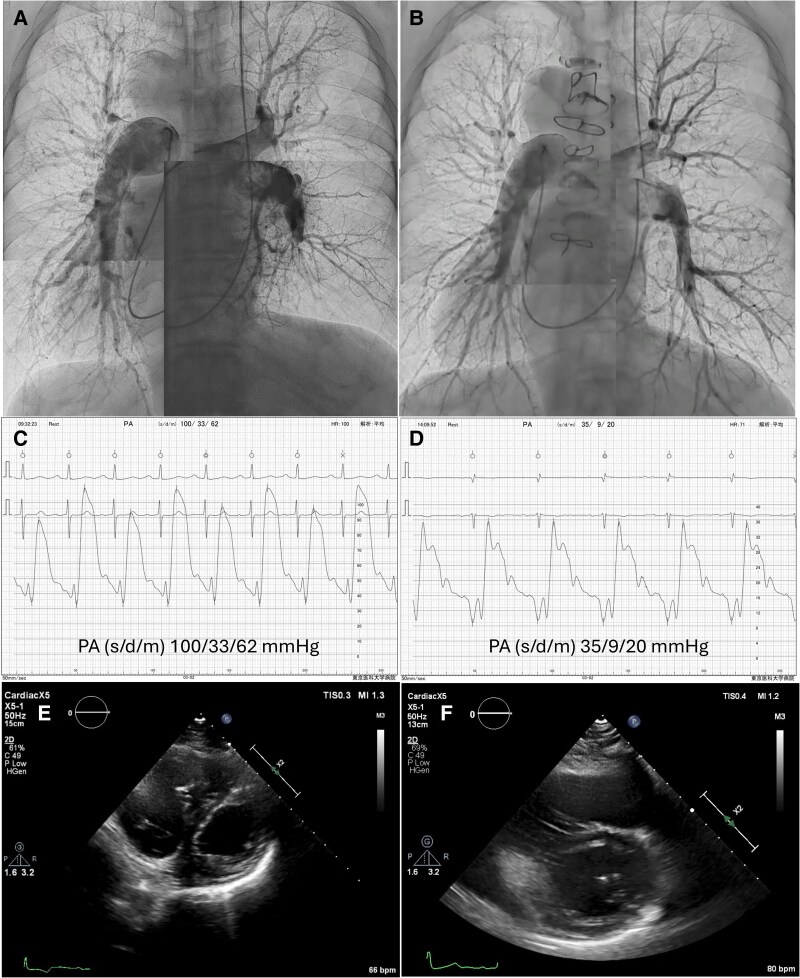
Pulmonary angiography, curve of pulmonary arterial pressure, and thoracic echocardiography before and after two balloon angioplasties and pulmonary endarterectomy. (*A*) Initial pulmonary angiography. (*B*) Pulmonary angiography after two balloon angioplasties and pulmonary endarterectomy. (*C*) Initial curve of pulmonary arterial pressure. (*D*) Curve of pulmonary arterial pressure after two balloon angioplasties and pulmonary endarterectomy. (*E*) Initial short-axis view in thoracic echocardiography when the patient lay down on right side. (*F*) Short-axis view in thoracic echocardiography after two balloon angioplasties and pulmonary endarterectomy. PA, pulmonary artery.
